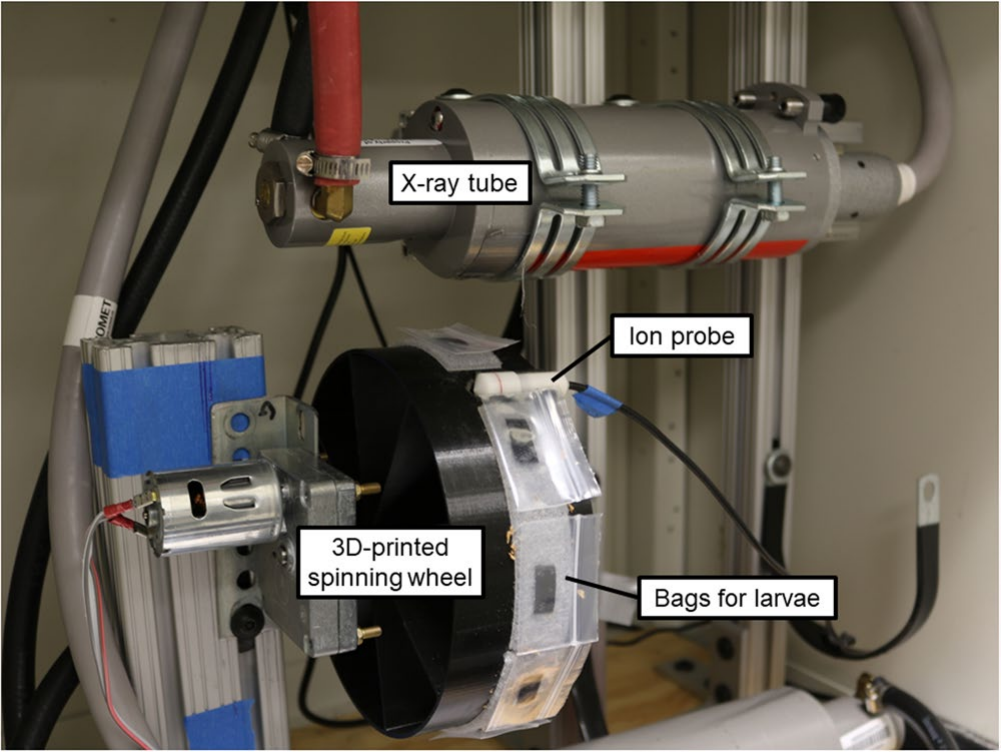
Configuration of the custom laboratory x-ray cabinet.
